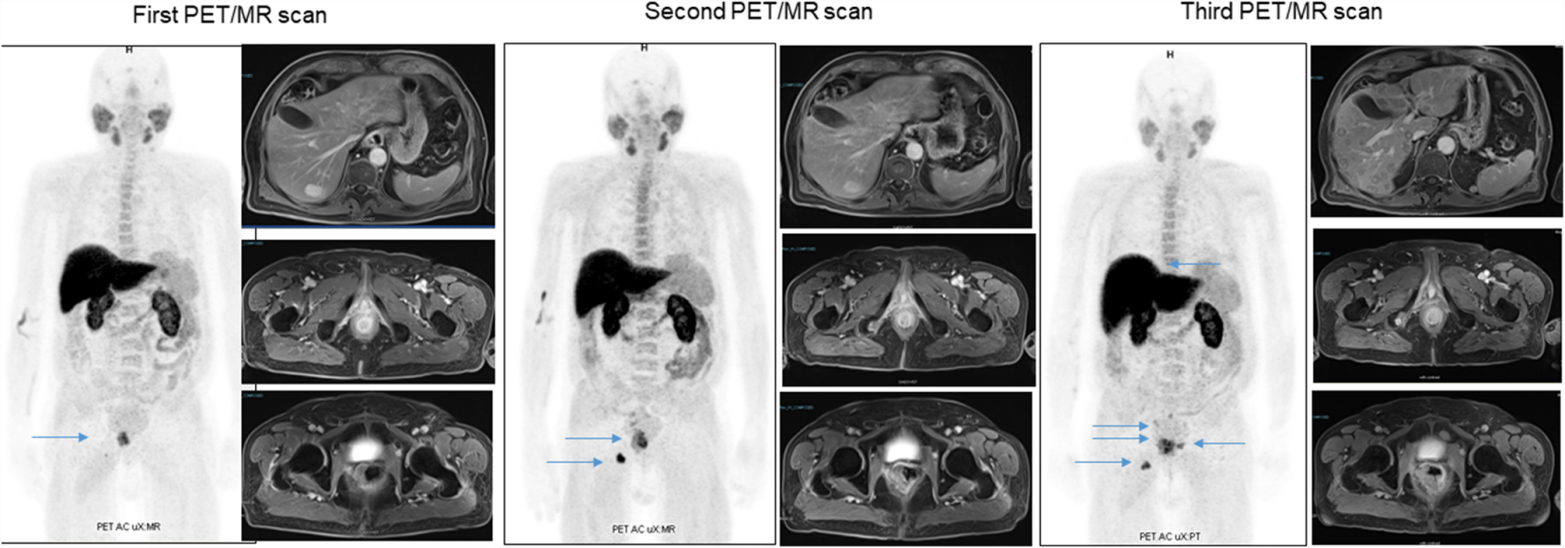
First, second and third [18F]F-choline PET/MR scan (maximum intensity projection on the left and transaxial fused images on the right, respectively), showing a para-rectal nodule (SUVmax 9, blue arrows) and bone (SUVmax 11.5 at the right ischium-pubic ramus) progressive disease, despite patient’s very low PSA values
